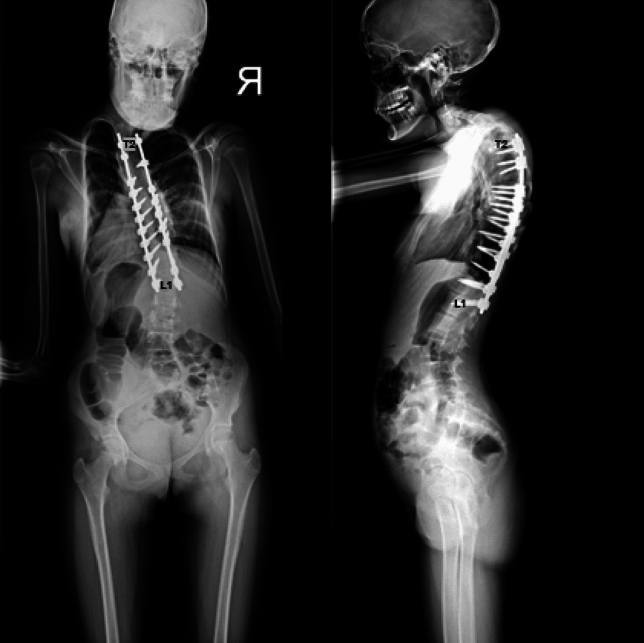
Case 1: Preoperative PA and lateral X-ray of a 12-year-old female with DiGeorge syndrome and syndromic scoliosis
